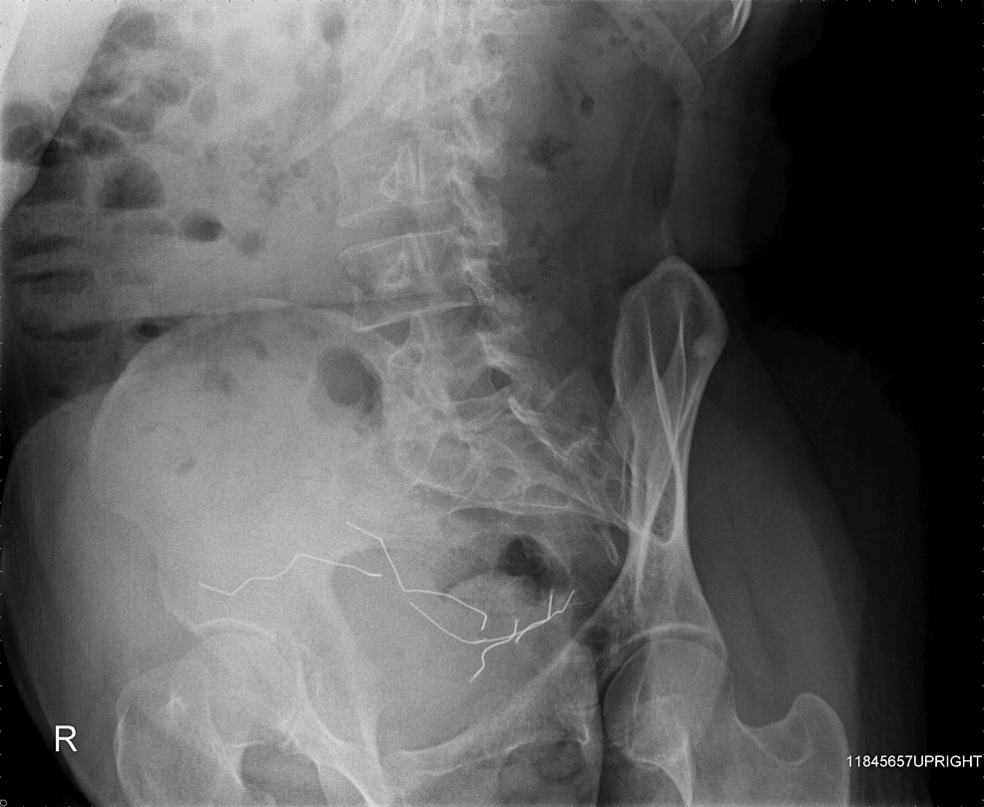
Lateral X-ray with paperclips embedded in the pelvic floor.
